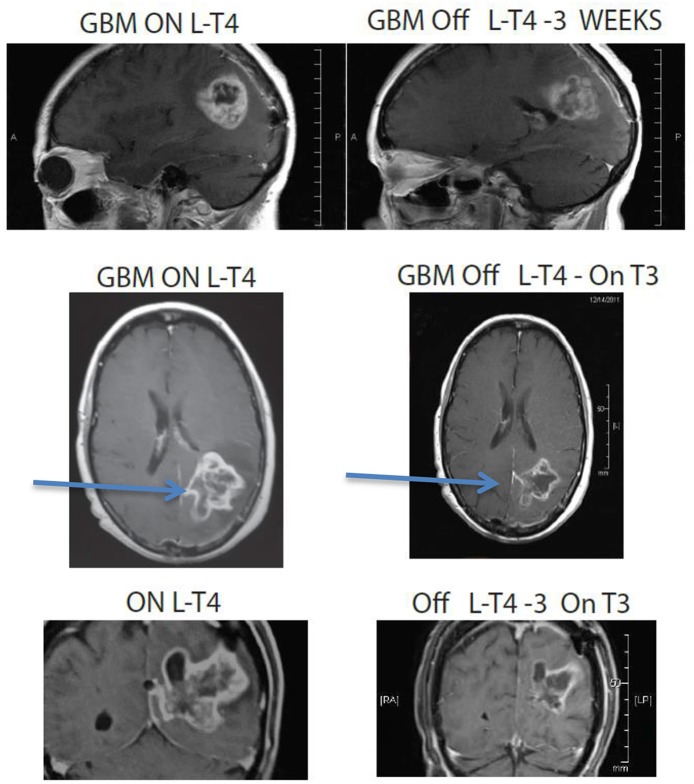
MRI images of a 67 year old female patient who was deteriorating neurologically with hemiplegia unresponsive to high dose dexamethasone. Discontinuation of L-T4 was followed within 1 week by significant clinical improvement and tumor regression. Arrows point to tumor mass, showing reduction in size in all dimensions following cessation of exogenous L-T4.
